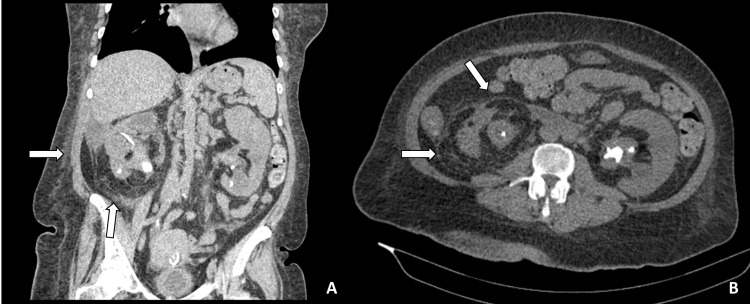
CT scan - (A) coronal section (B) transverse section showing the destruction of the right renal parenchyma with the extent of infiltration into the perirenal fat and retroperitoneal tissue
